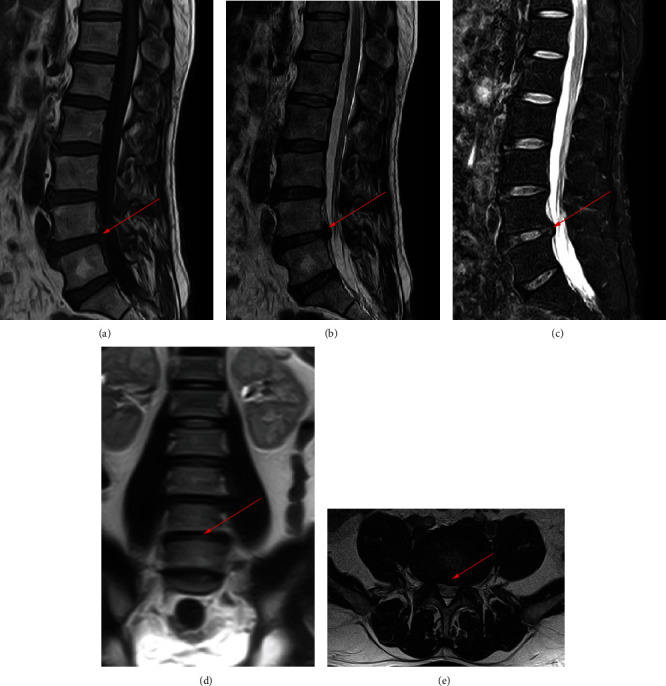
MRI examination of a patient with nonspinal tuberculosis. The arrow points to the location of the lesion. (a) MRI image in the sagittal T1 sequence. (b) MRI image in the sagittal T2 sequence. (c) MRI image of T2 lipid compression sequence in sagittal position. (d) MRI image in coronal position. (e) MRI image in cross-section.
